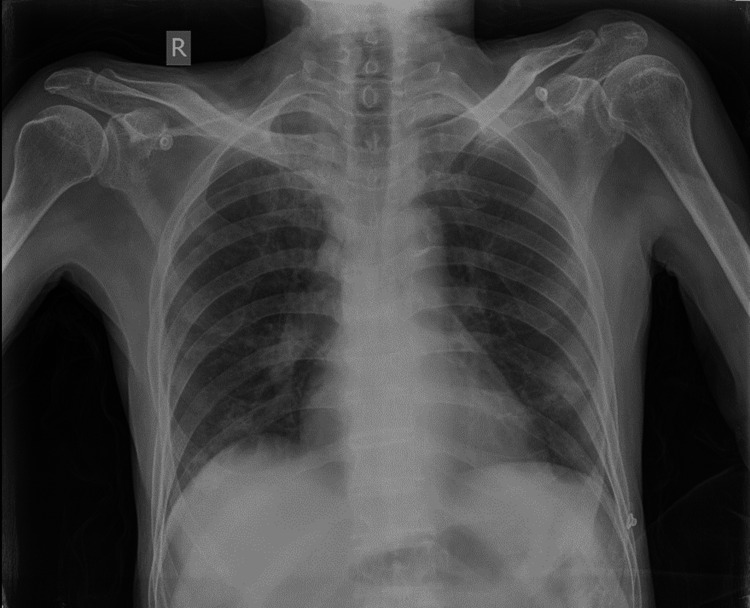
Chest radiograph showing no obvious abnormality.
